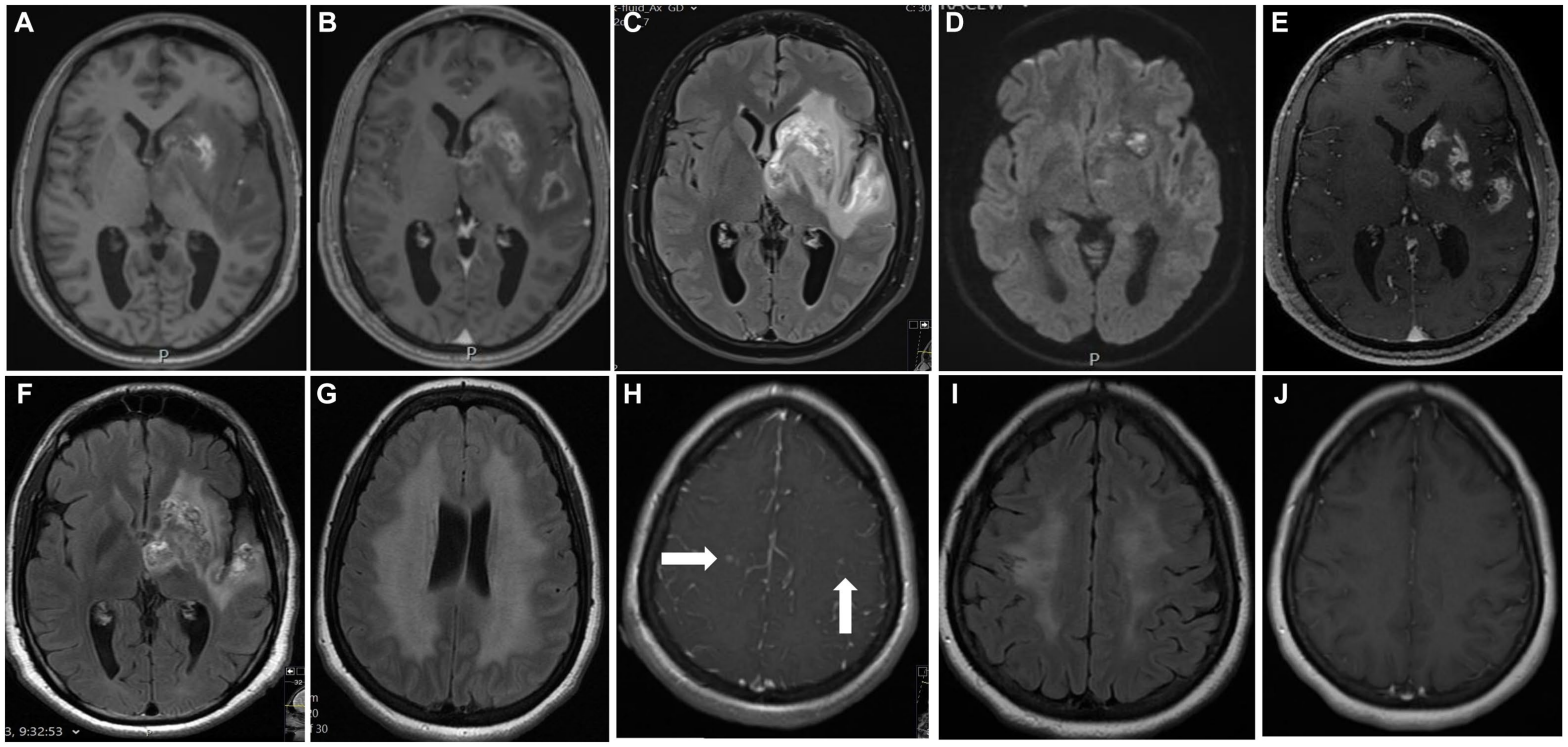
Main MRI features: Shown are selected figures for 2 of our 5 cases. The first case performed an MRI of the brain on admission (A–D) shows lesion with high signal on T1 weighted image at the basal ganglia (A) demonstrated areas of hemorrhage, with ring enhancement after gadolinium administration (B), vasogenic edema at the temporal lobe on T2FLAIR (C) and restricted diffusion on DWI (D) at both left basal ganglia and insular cortex. (E,F) demonstrate the MRI during treatment with decrease degree on enhancement (E) and vasogenic edema (F). Second case performed MRI on admission show symmetrical high signal on T2-FLAIR of the white matter at the corona radiata on both sides (G) with small foci of enhancement after gadolinium administration at the right centrum semiovale (H, arrows), and symmetrical areas of restricted diffusion on DWI of the centrum semiovle on both sides (NOT SHOWN). A consequent MRI after treatment show decrement of the high signal on T2-FLAIR (I), and no enhancement after gadolinium administration (J).
